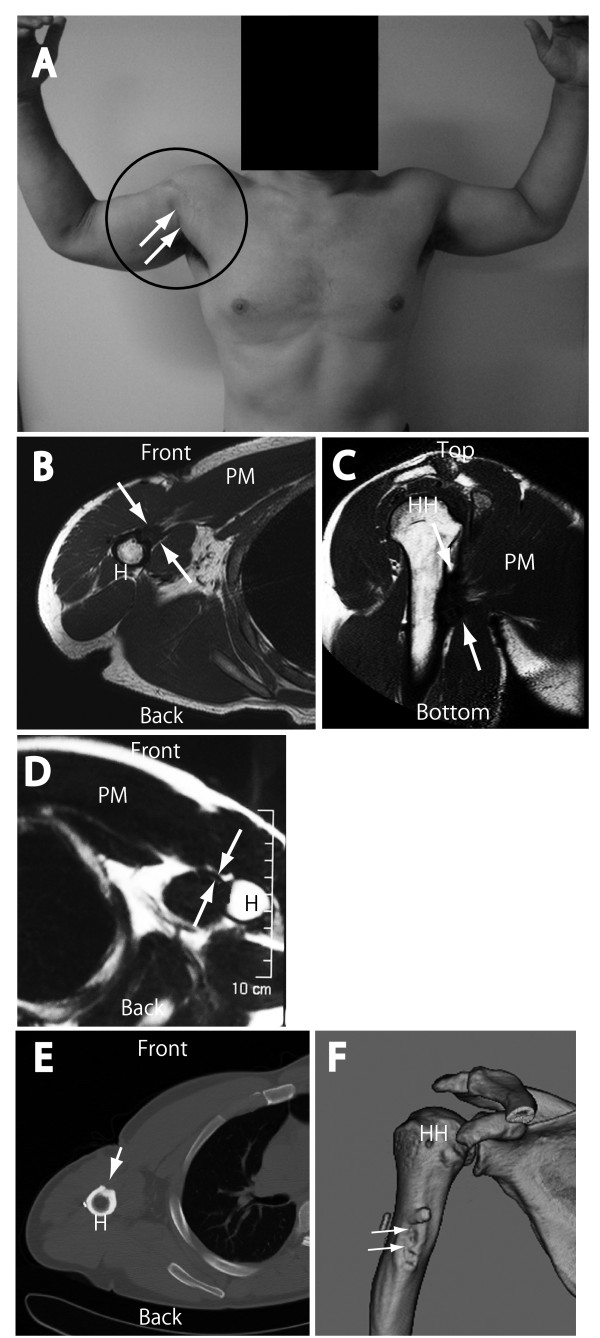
Postoperative external appearance and imaging in patient 1. The postoperative photograph shows that the loss of the axillary fold (contour of the anterior chest wall) had recovered 30 months after injury (A, white arrows inside the black ring). Magnetic resonance imaging (MRI) with axial (B) and coronal (C) views; spin echo T2-weighted image shows continuation of the tendon between the pectoralis major muscle and humerus (B and C, between white arrows). The thickness of the affected PM tendon (B, between white arrows) was significantly greater than that of the unaffected PM tendon (D, between white arrows). Furthermore, the rectangular bone trough was ossified as determined by computed tomography (CT) (E, white arrow) and three-dimensional CT (F, white arrows), and this bone union indicates that the reconstructed tendon was fixed strongly to the humerus. PM, pectoralis major; H, humerus; HH, humeral head.
